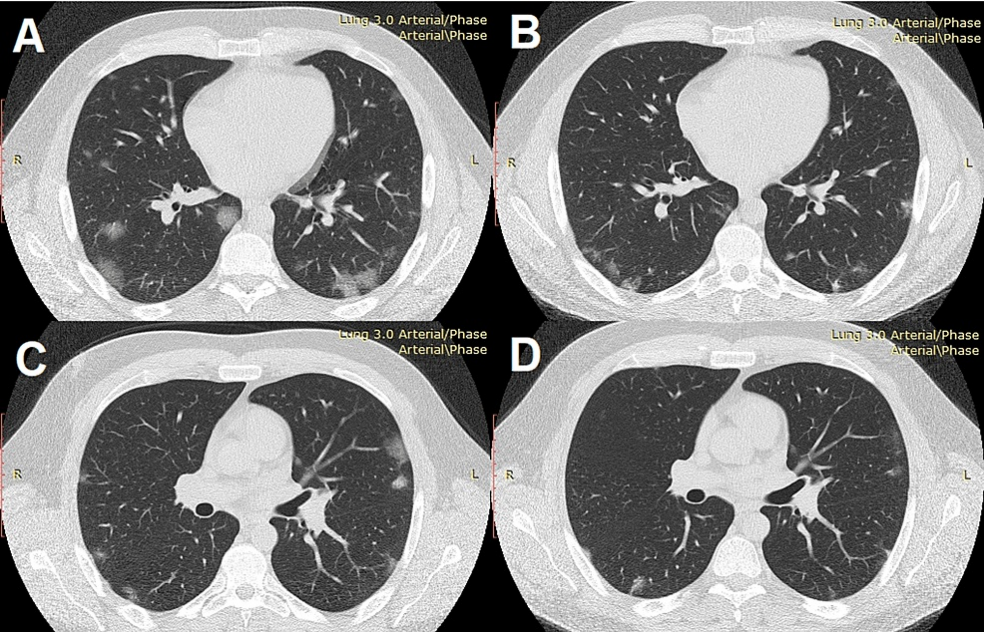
Dynamics of radiological changes in chest CT A and C - axial views with bilateral patchy ground-glass opacities and pericardial effusion B and D - axial views five days after initiation of therapy with reduction of pericardial effusion and ground-glass opacities CT: computed tomography
